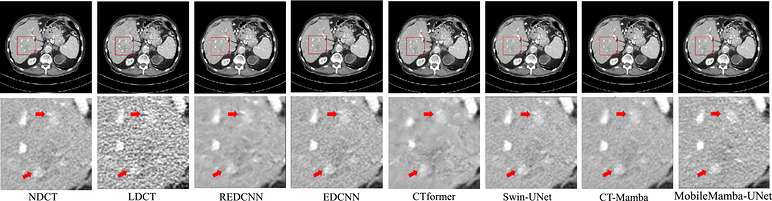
Detailed visual comparison on a randomly selected abdominal CT slice from the test set (displayed in the window of [‐160, 240] HU). Our MobileMamba‐UNet achieves better noise suppression while preserving fine details and tissue boundaries, producing results closest to the NDCT reference.
